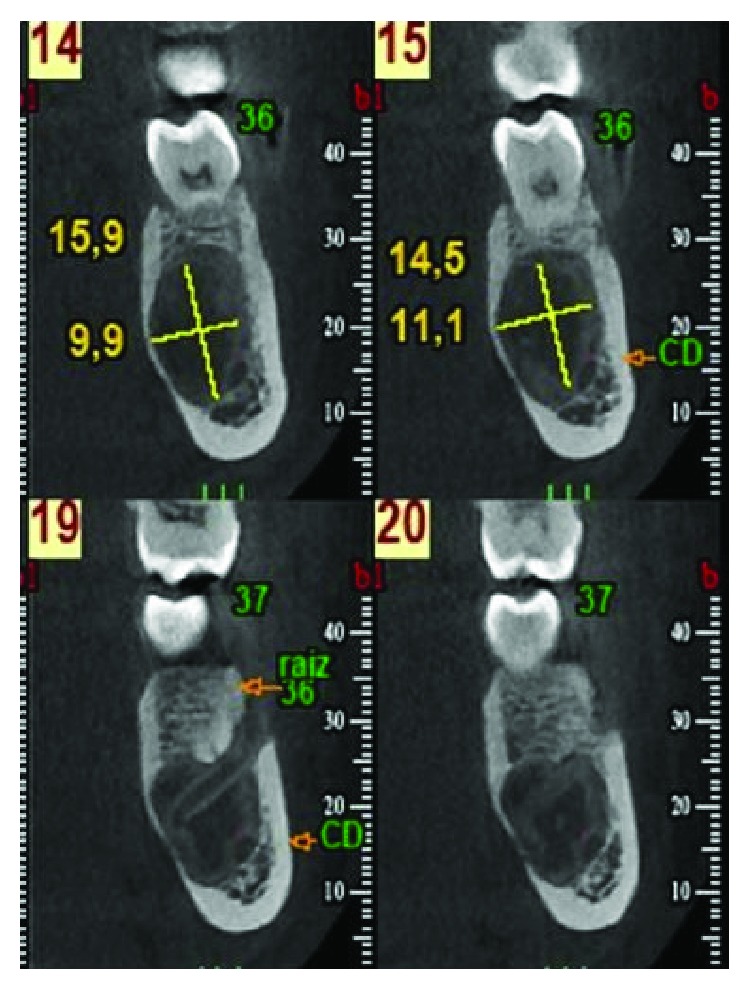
Cone beam computerized tomography 2 months after surgery. A slight reduction in lesion size (11.1 × 15.9 mm) is seen. Note the incipient recovery of the cancellous bones in the radiolucent image. The drainage catheter placed in the area of the lesion can also be seen.
